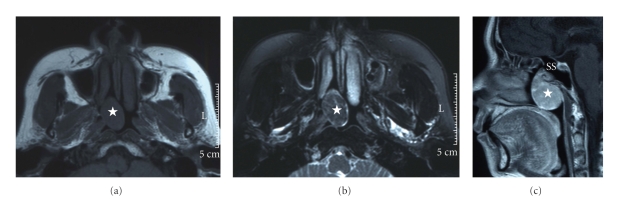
T1- (a) and T2-weighted (b) magnetic resonance (MR) images of the tumor (★). Gd-enhanced sagittal MR image (c) revealed that the tumor originated in the right sphenoid sinus (SS).
